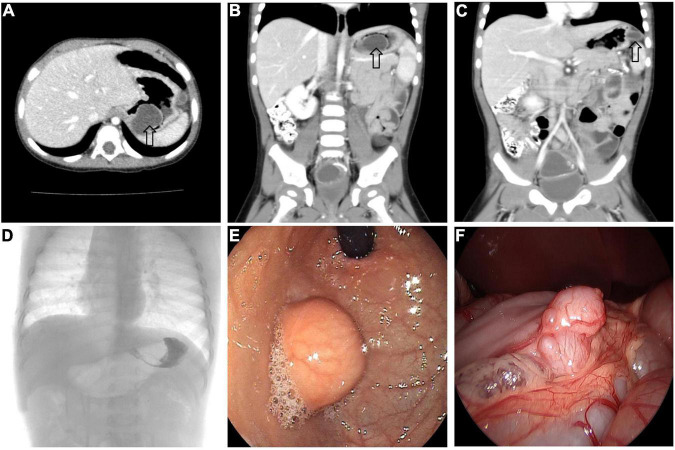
Multiple GDs (arrow) in a 1-year-old boy. (A–C) CT showed GDs located at the gastric funds and greater curvature. (D) Upper gastrointestinal radiography showed filling defect in the gastric funds. (E) Gastroscope found a spherical mass at the gastric fundus with a smooth surface. (F) An irregular mass can be seen at the great curvature of the stomach under laparoscopy.
